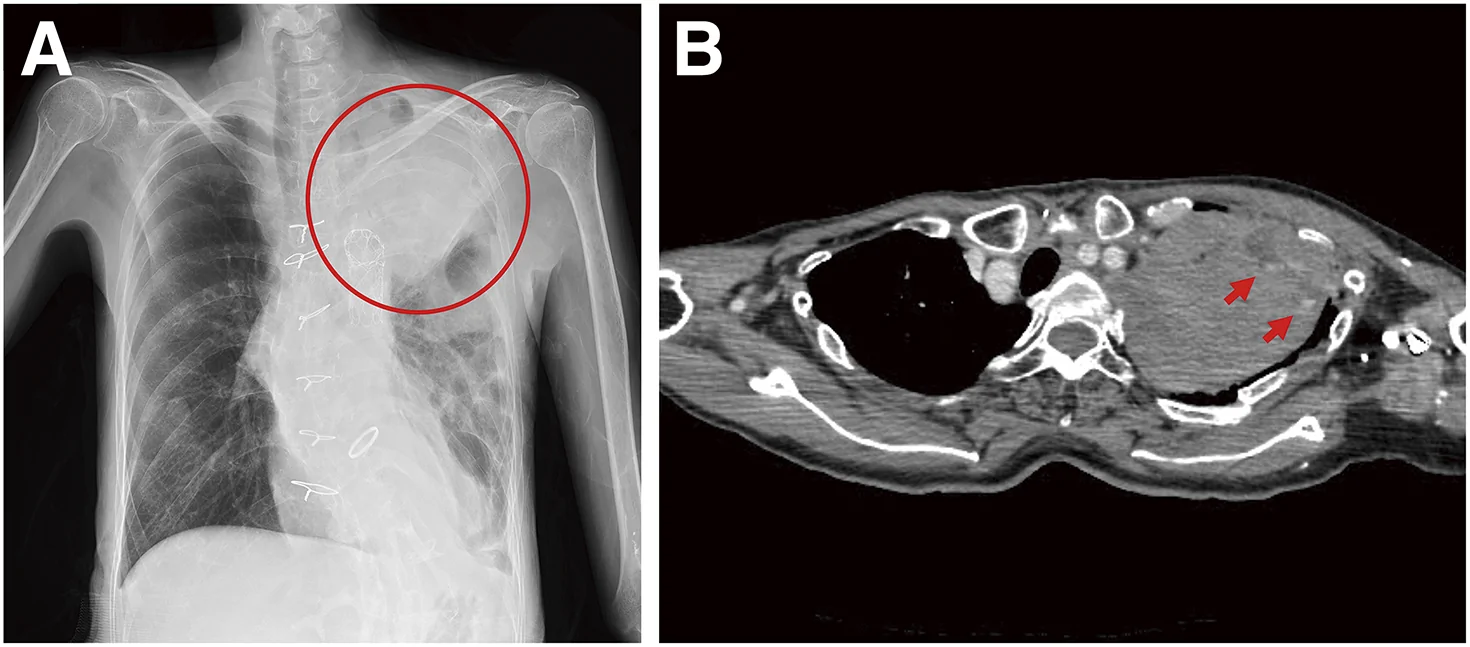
Imaging findings at admission. (A) Chest radiography showed a large opacity in the left hemithorax (circle). (B) Contrast-enhanced CT revealed a massive hematoma in the left thoracic cavity, with focal contrast enhancement (arrows) within the hematoma.
